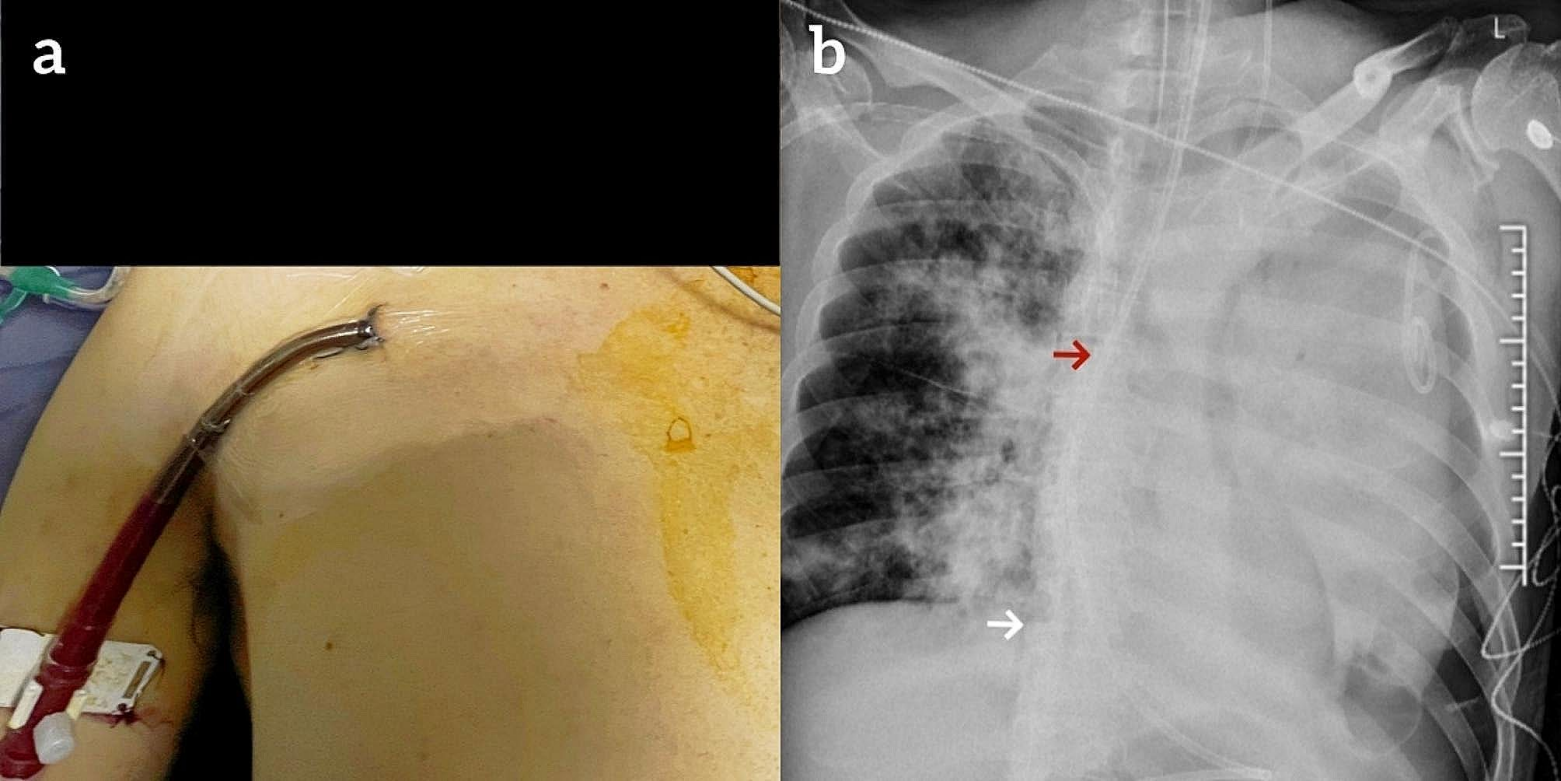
Axillary vein is punctured and inserted into the return cannula (a). Bedside chest X-ray shows the return cannula tip (red arrow) and the drainage cannula tip (white arrow) (b)
